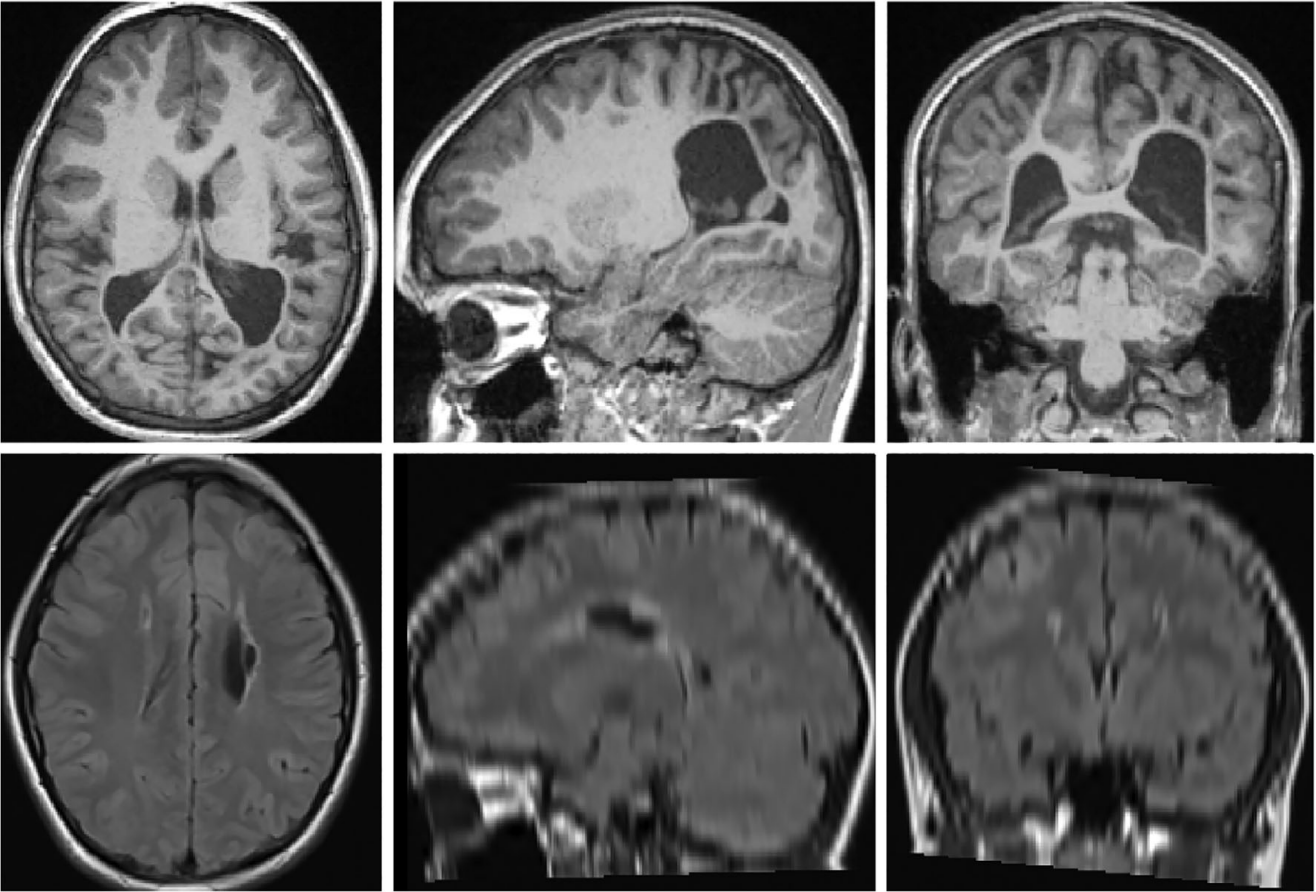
Axial (first column), sagittal (second column) and coronal views (third column) from the T1 and T2 weighted MRIs of two participants with CP classified with bilateral injury. Row 1 shows a participant with bilaterally enlarged ventricles and posterior cortical atrophy. Row 2 shows a participant with bilateral periventricular leukomalacia, visible as the hyperintense regions from the T2 TIRM sequence. Note that this sequence has a large slice thickness (5.2 mm), the resampled isotropic image appears blurry in both sagittal and coronal views. CP, cerebral palsy; MRI, magnetic resonance image; TIRM, turbo inversion recovery magnitude
